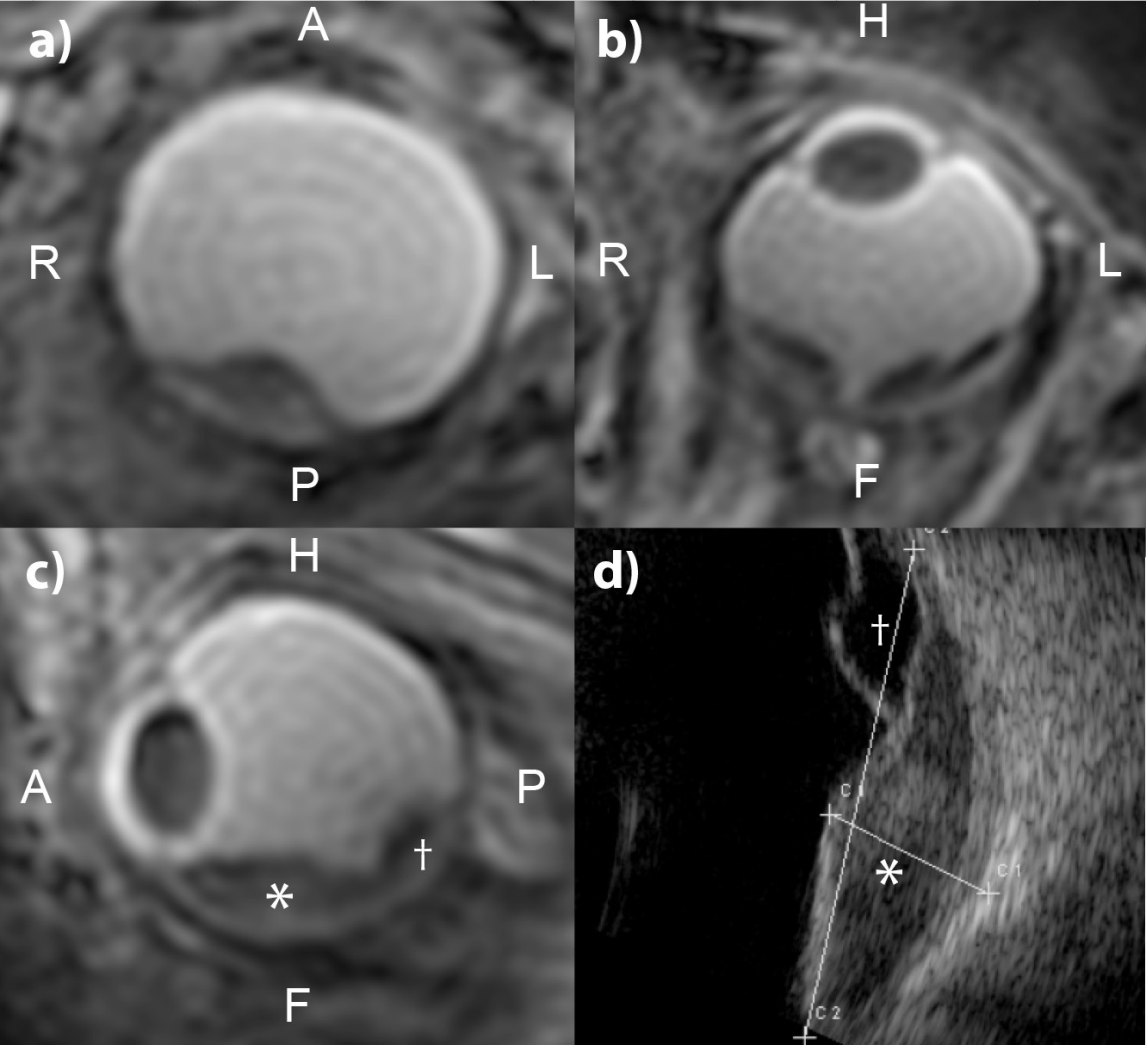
Reformatted high-resolution ZTEs and ultrasound frame for comparison of UM-patient 9. (a,b,c) Reformats of one T_2_-prepared ZTE scan in three orthogonal directions showing the complex three-dimensional shape of the lesion (marked by *) and retinal detachment (marked by †). (d) Ophthalmic ultrasound is only able to provide a 2D cross-section of the lesion (tumor extent marked by c1, c2 lines with crosses).
